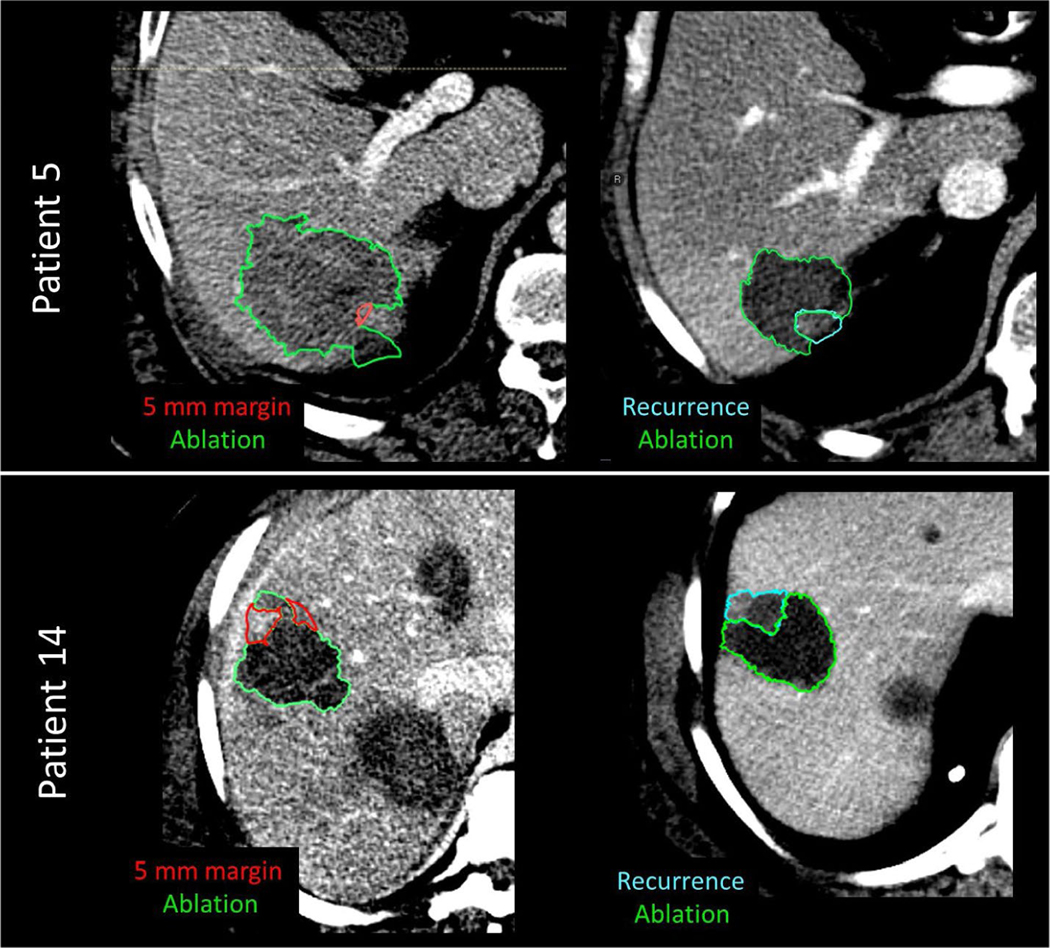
Example of 5-mm expansion on post-treatment CT images (left column) vs recurrence images (right column) for patient 5 and patient 14
